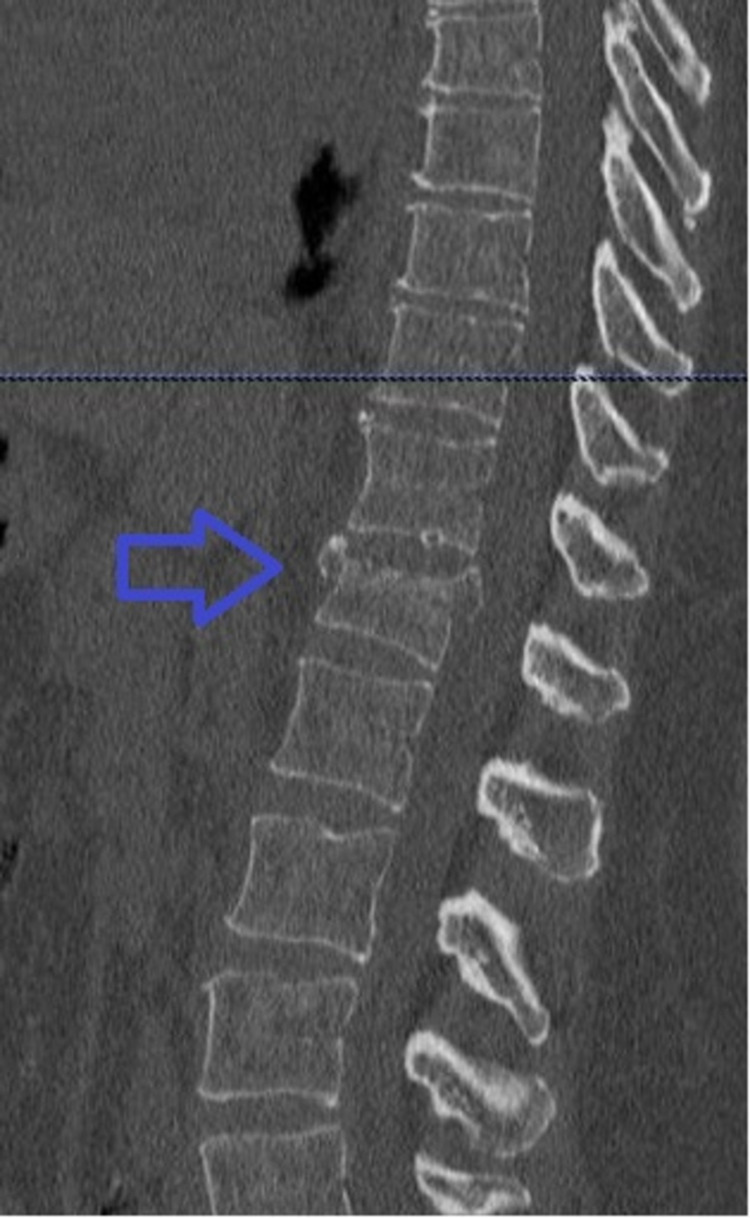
Saggital computed tomography reconstruction image. Blue arrow highlights the fracture of Th12 vertebrae.
